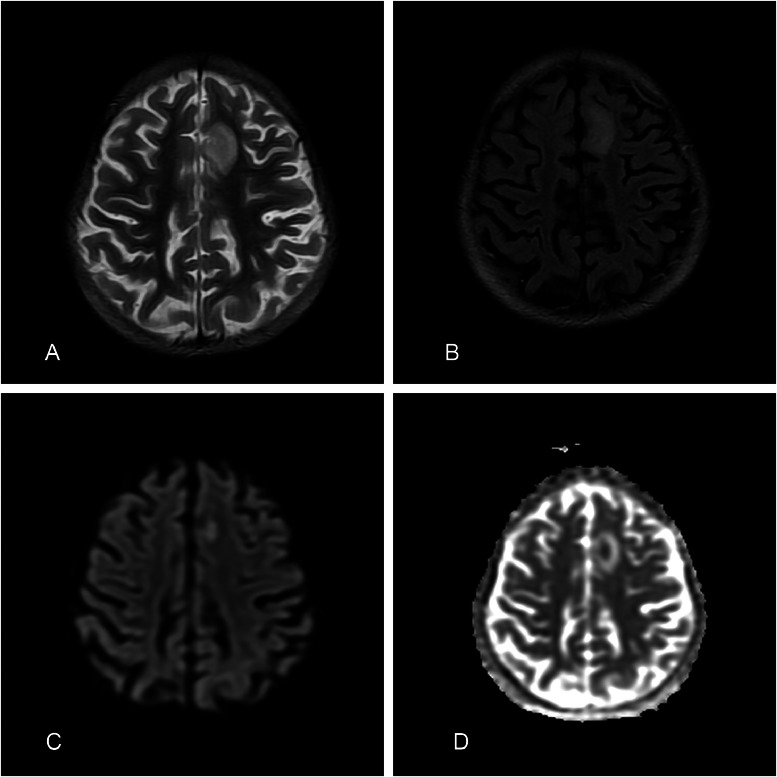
A focal altered signal intensity area is seen involving the cortex and subcortical white matter in left superior frontal gyrus. It appears hyperintense on T2/FLAIR and T2W (A, B), shows mild central diffusion restriction on DWI/ADC images (C, D), with no significant post contrast enhancement.
